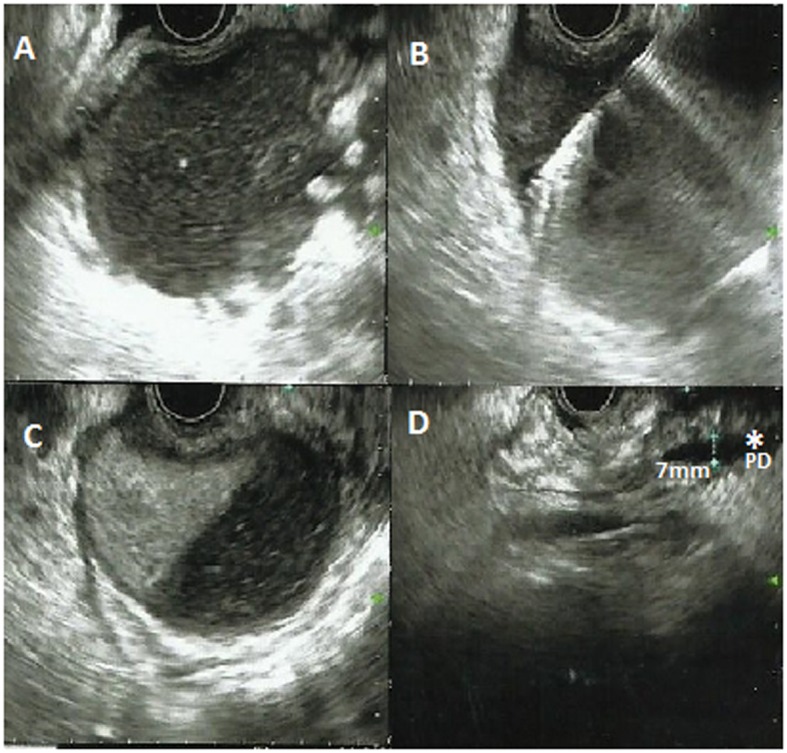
Endoscopic ultrasound revealed ill defined cystic lesion in the head of the pancreas with some solid component (A, B, C). EUS guided needle aspiration was taken (B). Pancreatic duct was dilated (7 mm) (asterisk) (D). The lesion was communicating with the second part of duodenum.
